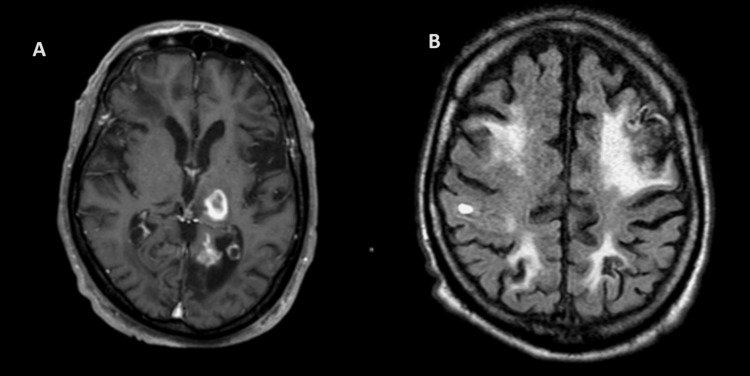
Magnetic resonance imaging of the brain on day 17 of hospitalization. A) T1-weighted post-contrast image in transverse view showing a ring-enhancing lesion in the left thalamus measuring up to 19 mm, with surrounding vasogenic edema; B) FLAIR image in transverse view shows nonspecific, wispy curvilinear enhancement within the bilateral frontal areas and stable enhancement in the left posterior temporal regions of encephalomalacia FLAIR: Fluid-attenuated inversion recovery
